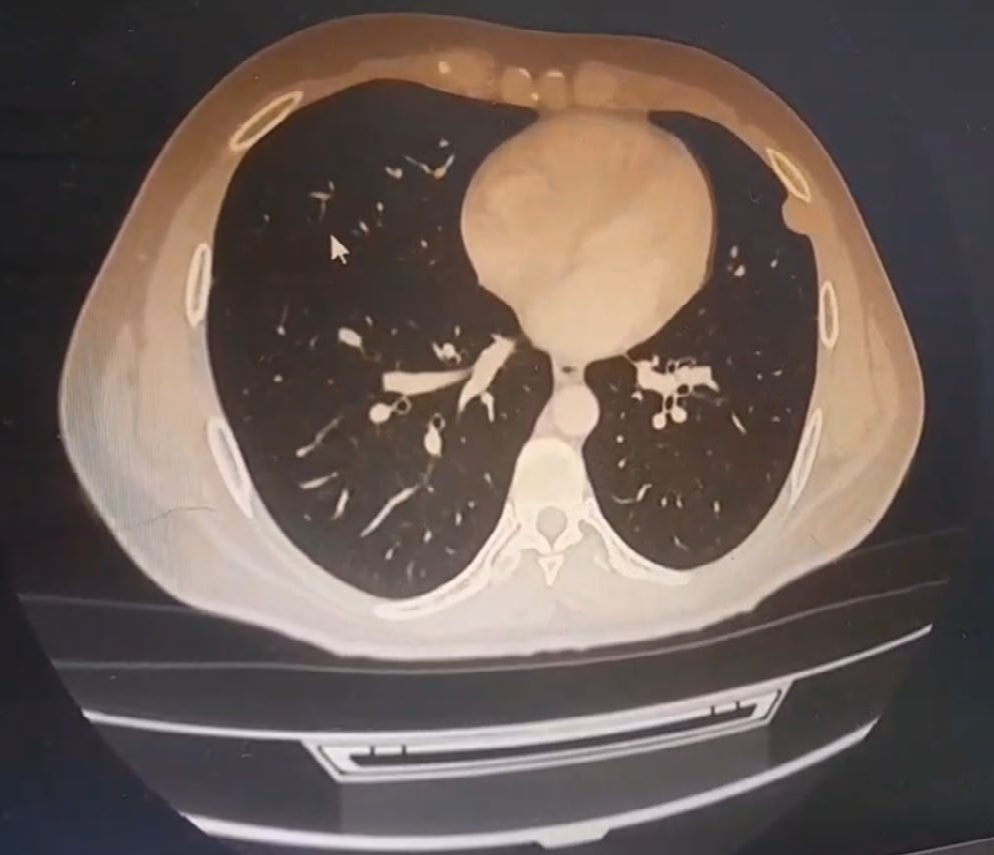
Axial chest CT (lung window) demonstrating a left pleural‐based subpleural lesion in the lateral segment of the lower lobe abutting the chest wall, without associated parenchymal consolidation.
